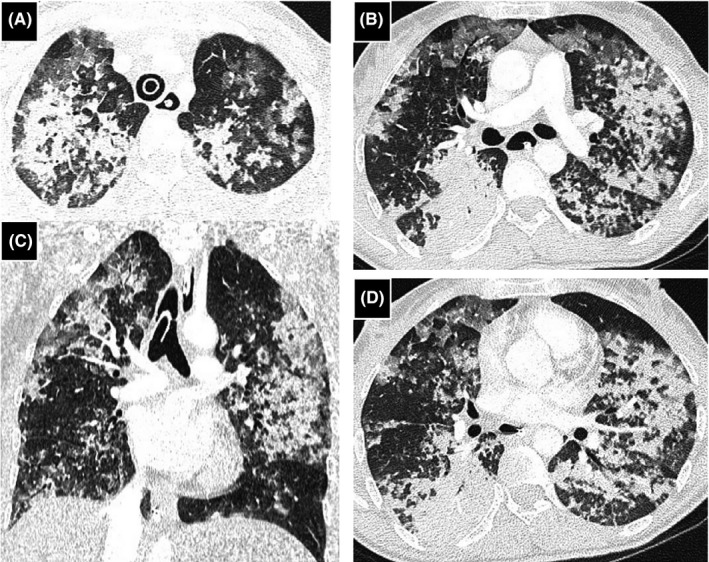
Chest computed tomography (CT) imaging, coronal (C) and axial (A, B, and D) views. Bilaterally multiple patchy ground‐glass opacities, associated with multifocal consolidations and septal thickening
